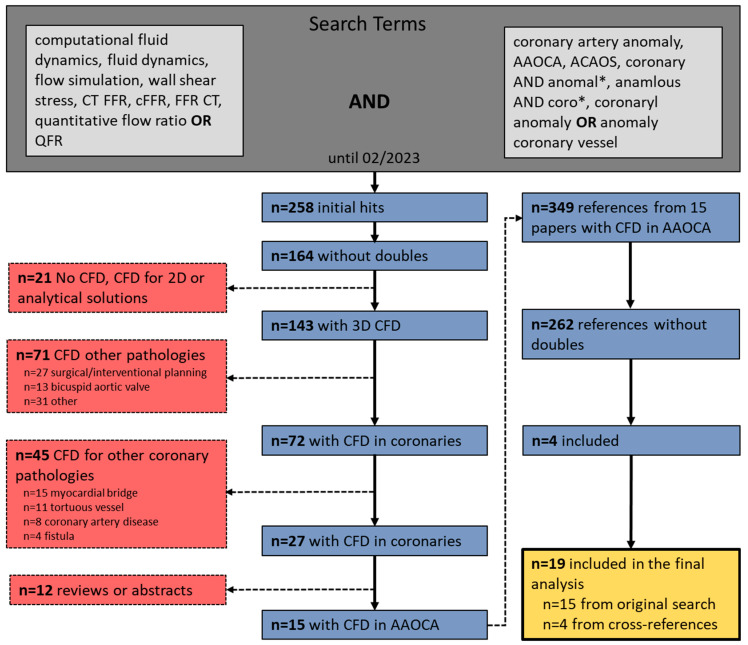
Consort flow of the study selection process. Abbreviations: CFD = computational fluid dynamics, CT FFR = computed tomography-derived fractional flow reserve, cFFR = CT-FFR from Siemens Healthineers, QFR = quantitative flow ratio, AAOCA = anomalous aortic origin of a coronary artery, ACAOS = anomalous coronary artery from the opposite sinus of Valsalva and * = wildcard symbol, that broadens a search by finding words that start with the same letters
